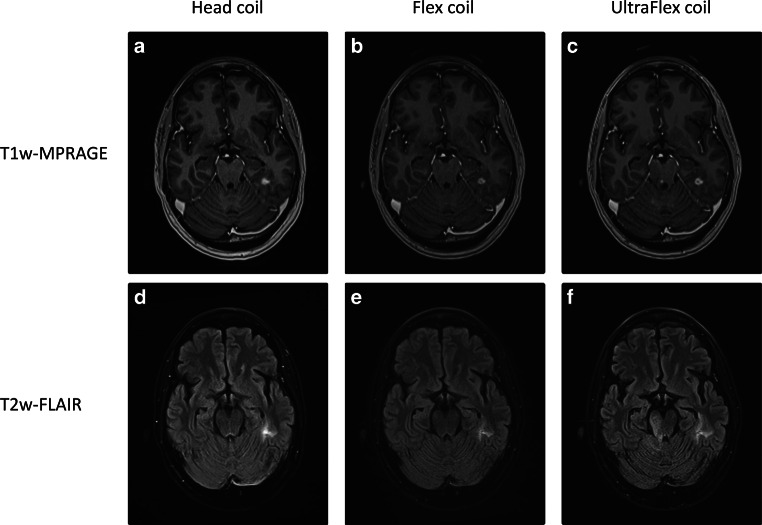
Images of a patient in all three investigated setups. a–c Images of the contrast-enhanced T1w-MPRAGE, d–f images of the T2w-FLAIR. a,b,d,e were acquired on the same day, c,f were acquired 77 days later. The contrast-enhancing lesion and the FLAIR-hyperintensity can be seen clearly in all three setups. While the Head coil shows relatively homogeneous signal-to-noise ratio, the noise in both mask setups increases significantly in anterior–posterior direction
